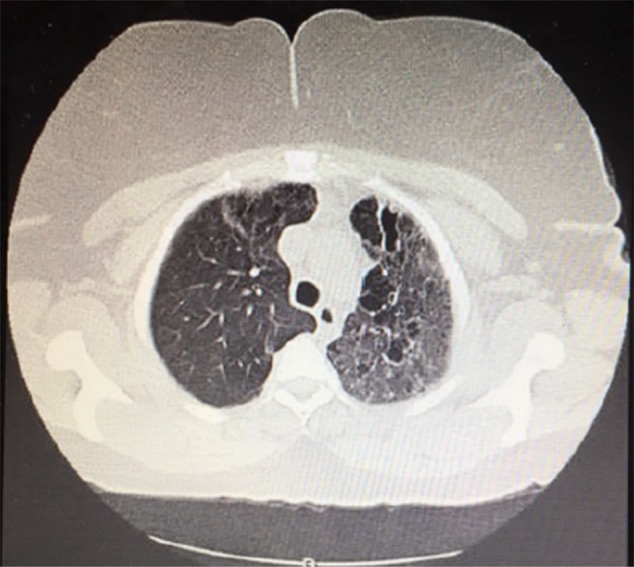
Computed tomography of the chest from our patient, revealing cystic bronchiectasis and ground-glass opacities in the left lung.
